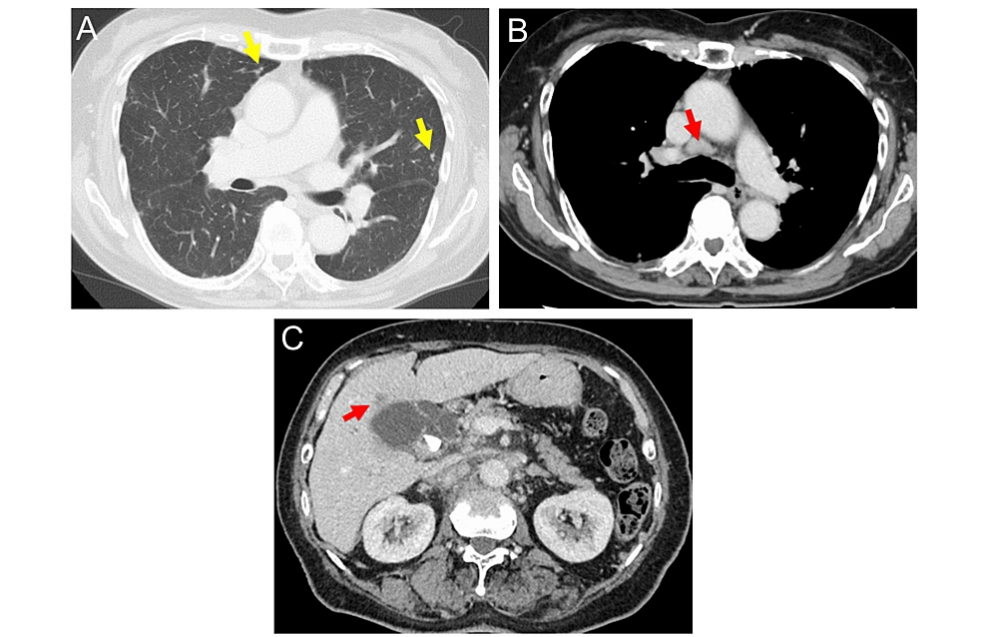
Computed tomography one month prior to admission A) The yellow arrows indicates multiple granular shadows. B) The red arrows indicate enlarged mediastinal and hilar lymph nodes. C) Computed tomography shows thickened gallbladder wall, visible gallstones, and a liver mass. The red arrow indicates the liver mass. A layer is observed between the liver mass and the gallbladder.
